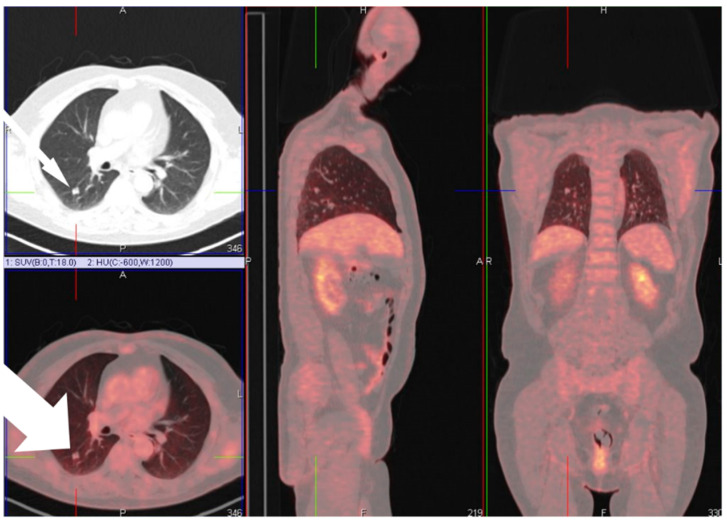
PET-CT showing moderate FDG-avidity of a lesion on PET scan in the right lower lobe with evidence of calcification or ossification on the diagnostic CT. FDG, fluorodeoxyglucose.
